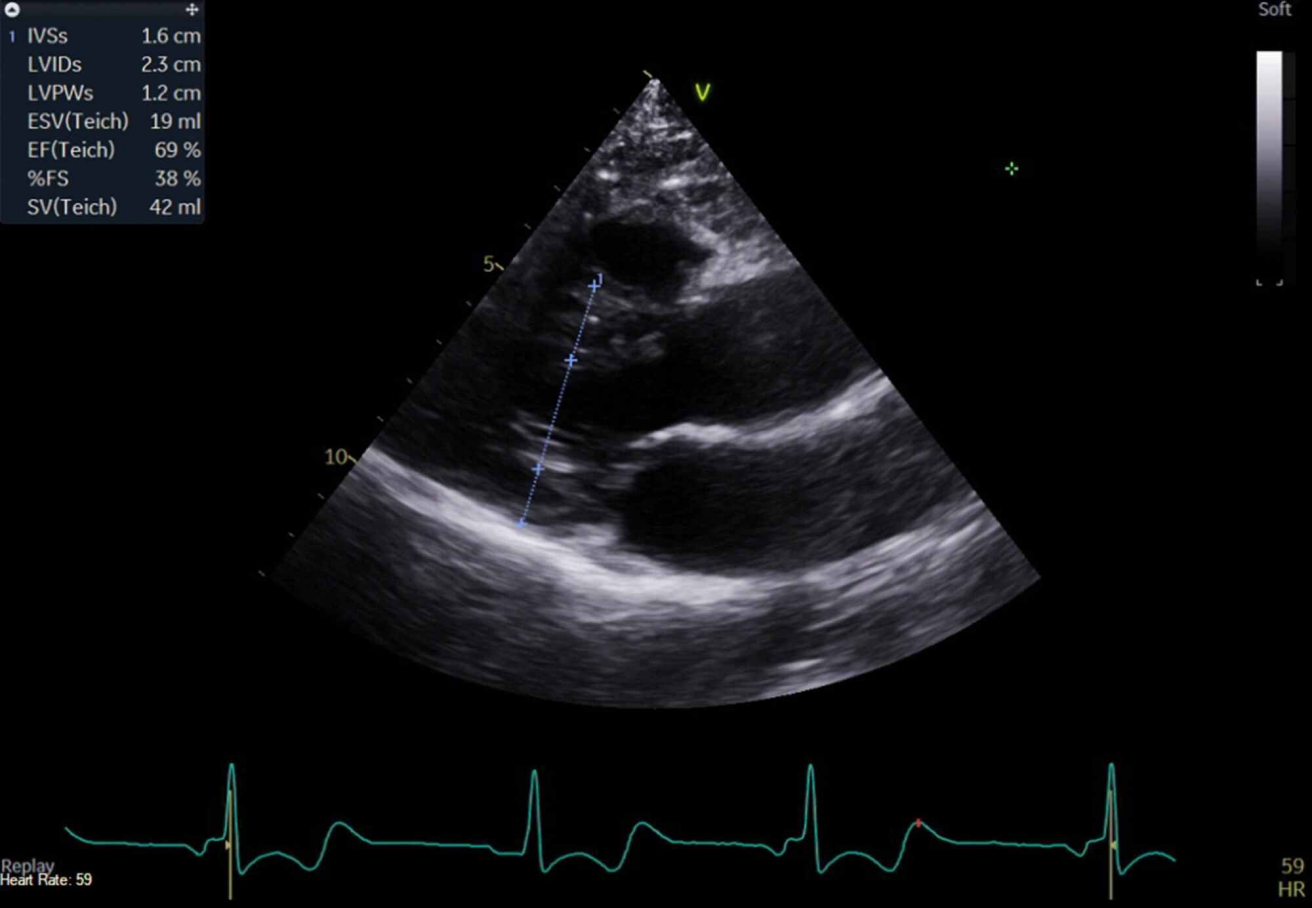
Echocardiogram demonstrating moderate asymmetric septal left ventricular hypertrophy with ejection fraction of 68%, wall motion was within normal limits, peak and mean left ventricular outflow tract (LVOT) gradients less than 4 and 2.5 mm respectively with no increase in gradient with provocative maneuvers such as Valsalva. Intraventricular septum measuring 1.6 cm, but mass located in the basal anterior wall.
